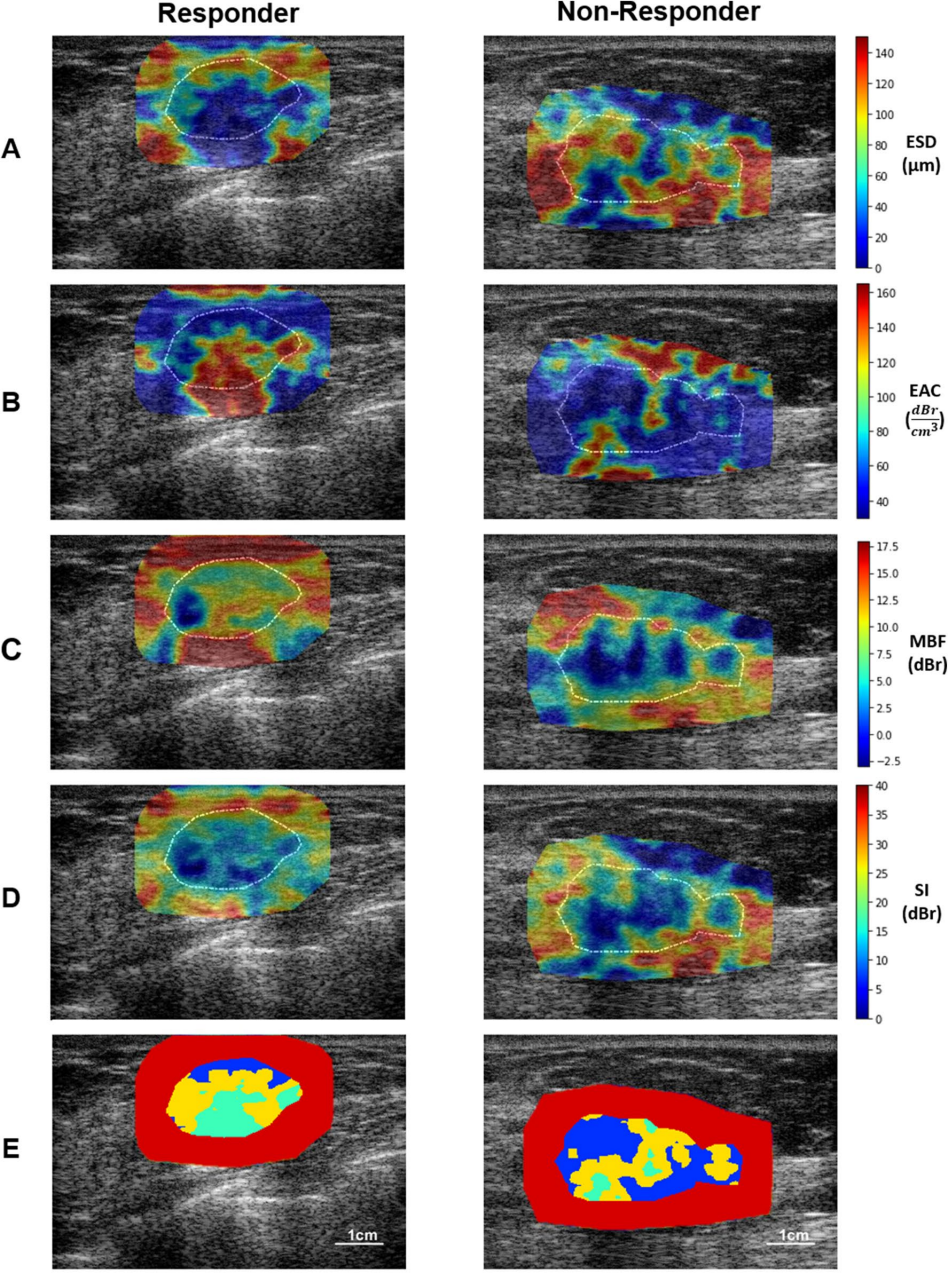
A-D: Ultrasound B-mode images with parametric overlays of ESD (A), EAC (B), MBF (C), and SI (D) acquired from a representative responder and non-responder to NAC. The tumor core has been outlined with white dashed line. (E) distinct intra-tumor regions (region 1: green, region 2: yellow, region 3: blue) segmented using the HMRF-EM algorithm, surrounded by the tumor margin area (red).
